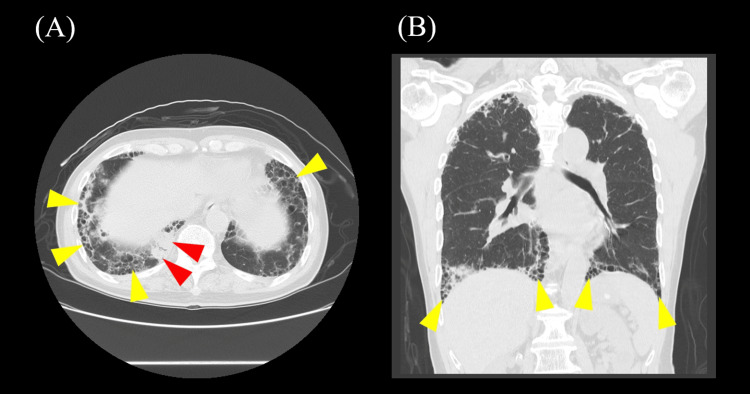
Results of the chest-abdominal computed tomography (CT) at age 73. (A, B) Chest-abdominal CT showing subpleural interstitial opacities and honeycombing in both lungs (yellow arrowhead), infiltrates in the right lung base (red arrowhead). (A) Axial section; (B) coronal section.
